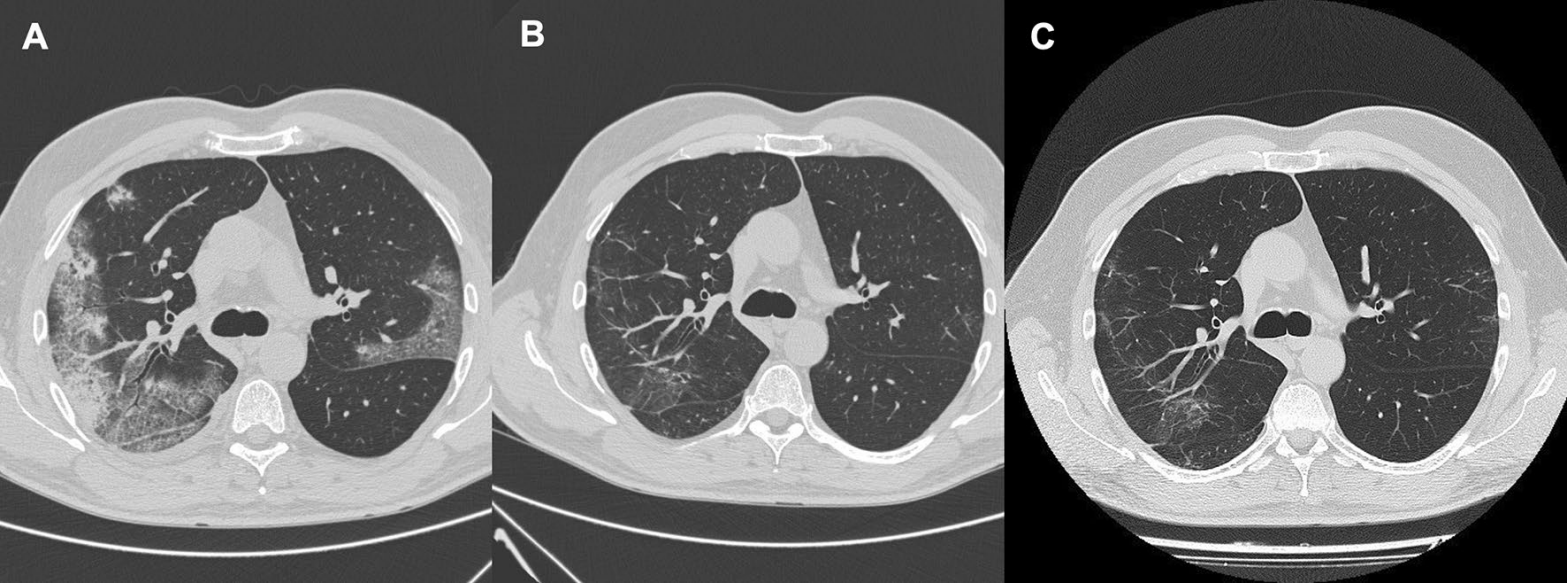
A Axial CT showing bilateral ground-glass and consolidative opacities and interlobular septal thickening. B At 6 months there is a reduction of ground-glass and consolidative opacities and of interlobular septal thickening. C At 12 months there is a resolution of ground-glass and consolidative opacities and of interlobular septal thickening and the appearance of subpleural curvilinear opacities
